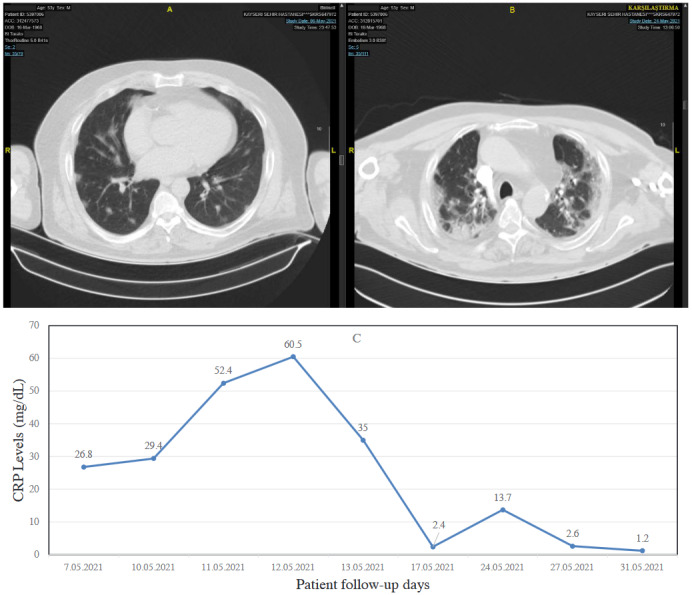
A sample for “severe disease” related to COVID-19. Thoracic CT imaging and CRP course of a 53-year-old male COVID-19 patient with hypertension and coronary artery disease as comorbidities. A) CT imaging at admission had a fever and CRP: 26 mg/dL, commenced dexamethasone at 2 × 8 mg/day. B) Progression of CT imaging on the 9th day of hospitalization. A single dose of 80 mg tocilizumab and dexamethasone 2 × 8mg/day for 10 days were ordered. C) CRP course of the patient.
